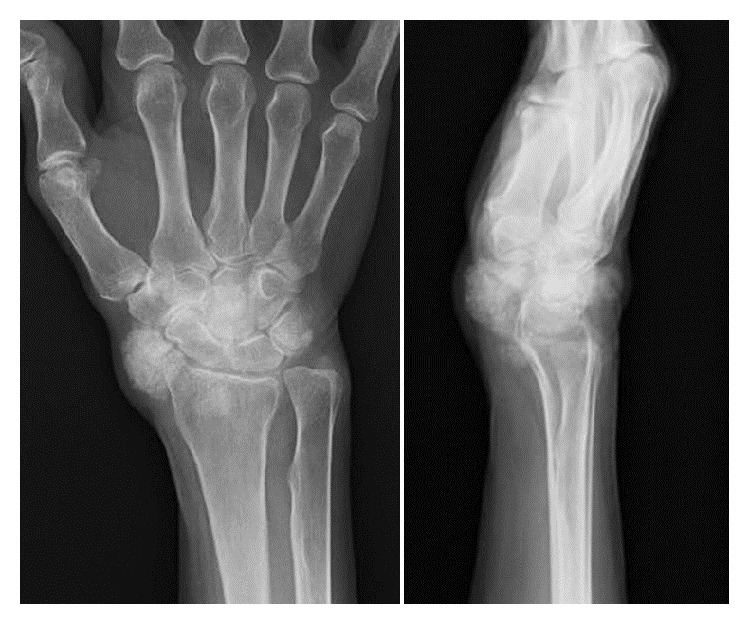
X-ray findings show some calcified nodular lesions on the right wrist joint.
